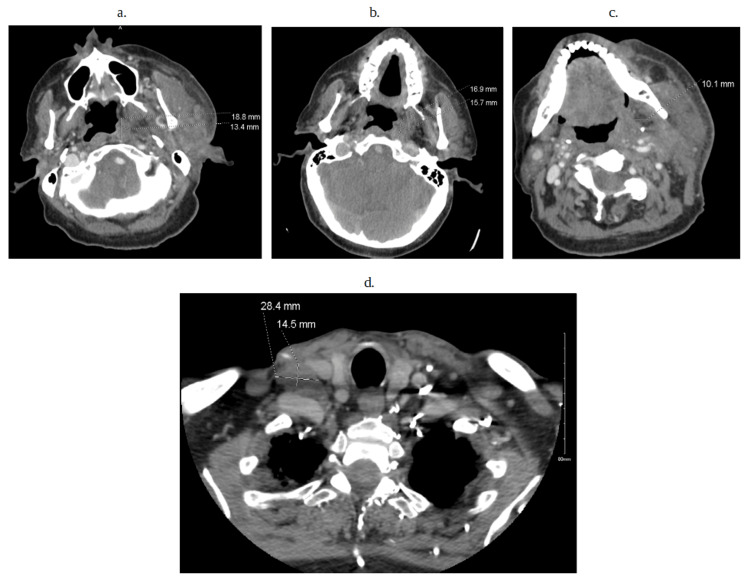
Computed tomography of the head and neck Figure 2a: shows an 18.8 x 13.4 mm nodule of the face on the left side of the fossa of Rosenmuller. The possibility of metastatic disease should be considered, given the possibility that a second primary left neck mass can’t be excluded. Figure 2b: shows a 16.9 x 15.7 mm enlarged lymph node. Figure 2c: shows that there is no evidence of enhanced encapsulated collection to suggest a frank abscess, but there is a 10 mm low attenuation collection in the left submandibular space that could represent a small phlegmon. Figure 2d: shows a 28 x 14 mm right superclavicular lymph node.
